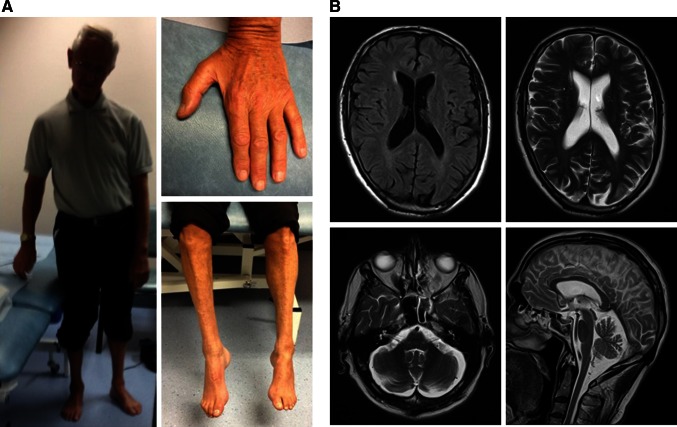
a Photographs of the patient demonstrating a partially treated torticollis, a broad-based stance, distal upper and lower limb atrophy as well as bilateral pes cavus. b MRI of the patient’s brain demonstrating cerebellar atrophy
